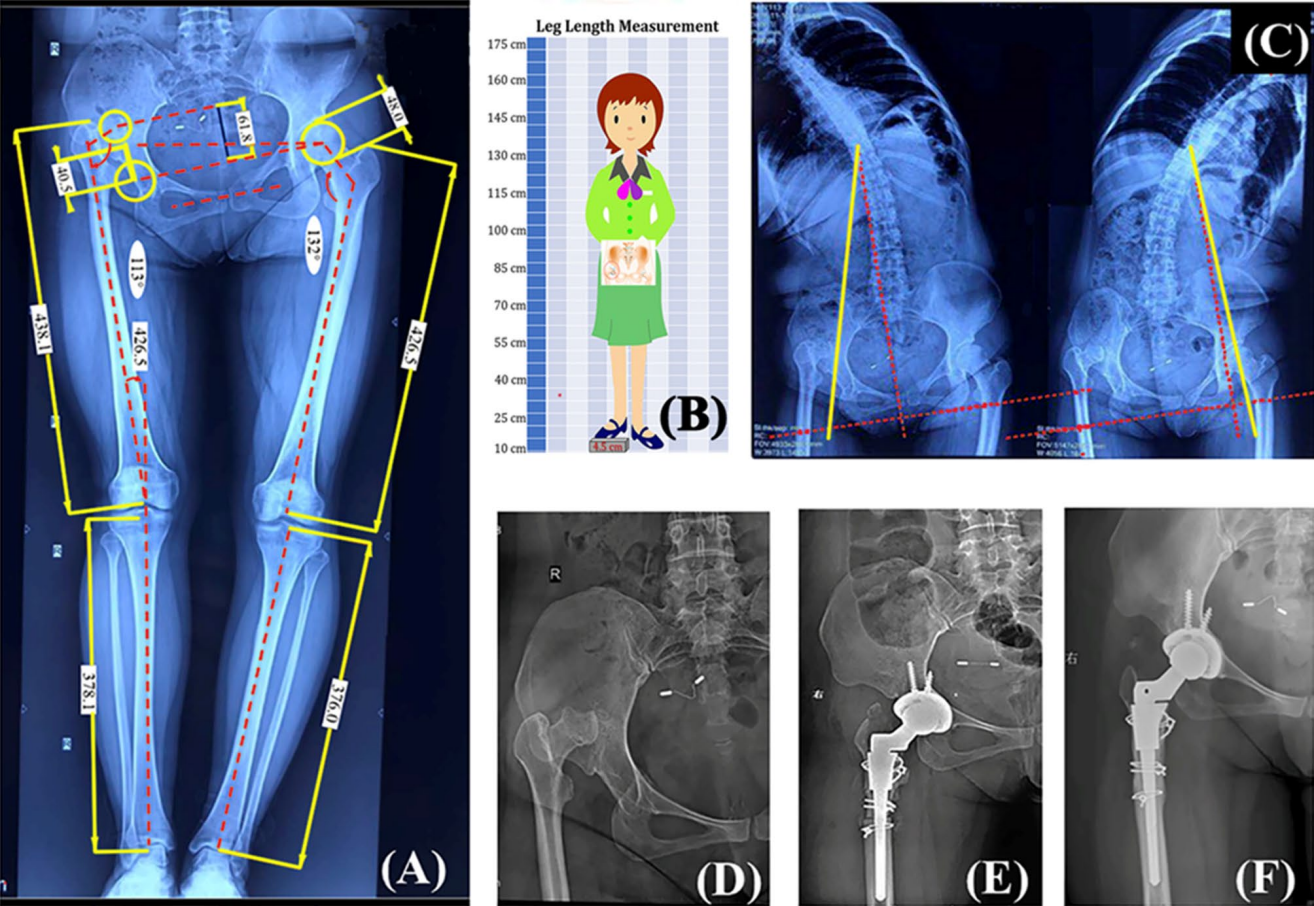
Preoperative planning and surgical treatment of a 37-year-old male patient diagnosed as Crowe type IV DDH by DAA THA combining STO. (A) Preoperative planning and lower limb length balancing by standing full-length anteroposterior radiographs. A stepwise algorithm for limb length equalization was implemented based on measurements of the hip dislocation height, the anatomical lengths of the lower limbs, the pelvic tilt angle, intra-articular and extra-articular deformities as indicated. (B) The limb length discrepancy was further evaluated by a scaled block under the ipsilateral foot in our measuring system. (C) Bilateral bending X-ray of lumbar spine were obtained to estimate the flexibility of the lumbar-pelvic complex. (D,E) Preoperative and postoperative anteroposterior hip radiographs. This patient was further balanced by STO within direct anterior approach (osteotomy length, 25 mm). (F) Osteotomy union at 7 months after surgery.
